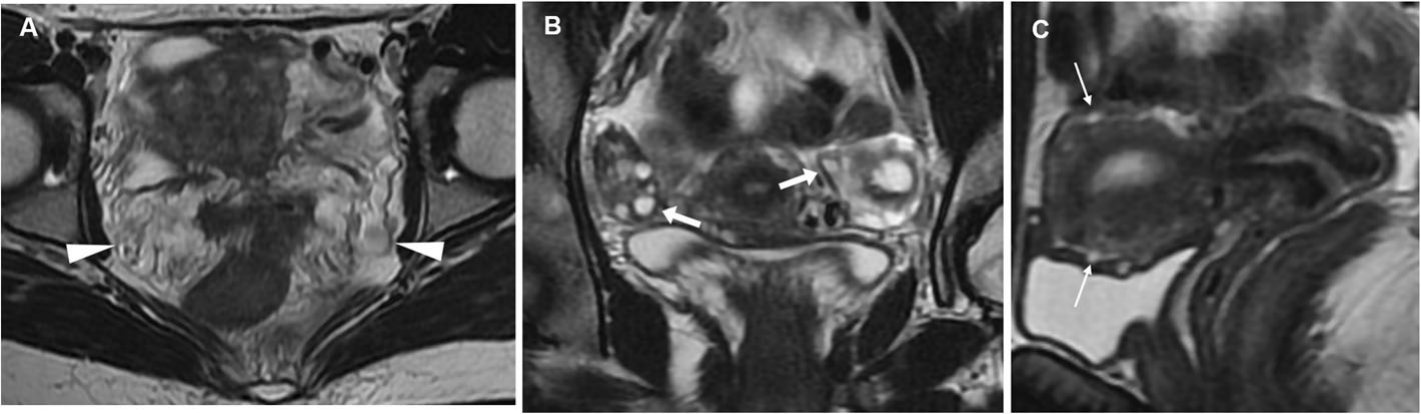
In the same patient as in Fig. 22, multiplanar T2-weighted images demonstrate severely congested pelvic veins (arrowheads in a), cystic components in both ovaries (arrows in b), and dilated arcuate veins in the subserosal portion of the myometrium (thin arrows in c)
